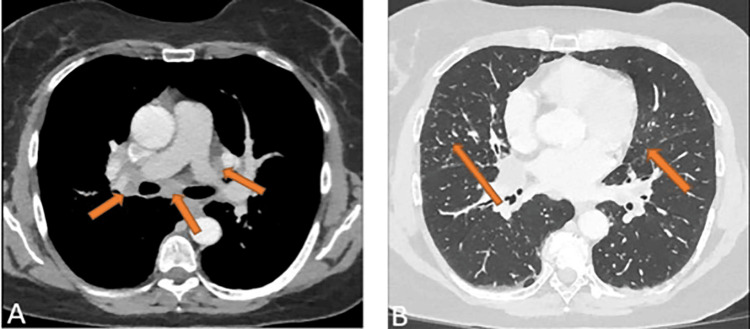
Thoracic CT at the ninth-month follow-up after the start of therapy (axial view). A. Compared to the previous CT scan, mediastinal and hilar lymph nodes (arrows) are smaller on both sides. B. Reduction in bronchial thickening, interlobular septal thickening, and subpleural consolidation (arrows).
